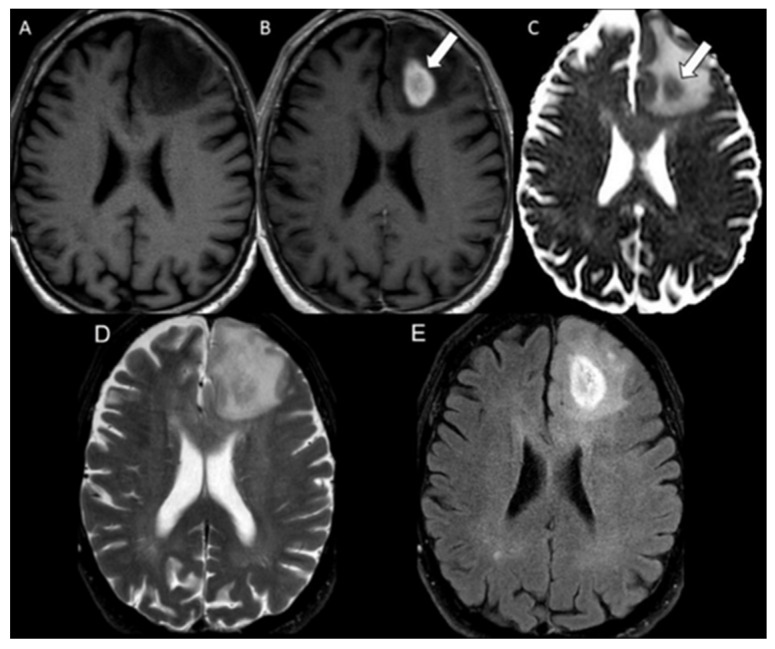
An 86 y/o male with tumor progression. Axial MRI of the brain including (A) T1 weighted image, (B) postcontrast T1 weighted image, (C) ADC map, (D) T2 weighted image, and (E) FLAIR. The lesion demonstrates central contrast enhancement in the left frontal lobe (white arrow in (B)) with corresponding restricted diffusion on the ADC map (white arrow in (C)) and areas of surrounding T2/FLAIR hyperintensity (D,E).
